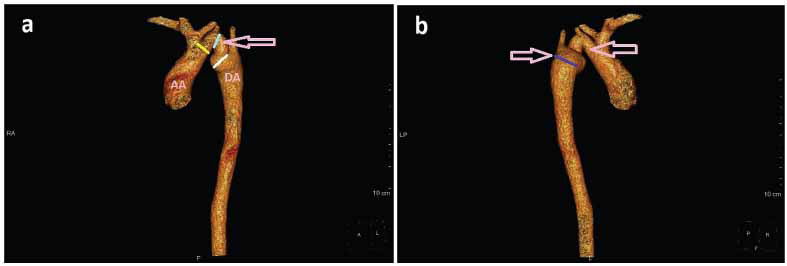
CT-image: 3-dimensional reconstruction of the ascending, arch, and descending aorta. The distal portion of the arch shows slightly reduced calibre, with redundancy and buckling. The descending aorta immediately distal to the buckled segment shows mild prominence in calibre, (a) Yellow line-Proximal arch measuring 2.6 cms, Light blue line-calibre of the kinked segment 2.4cms, and white line- the calibre of the distal portion of the aortic arch, 2.7cms. The pink arrow shows the kinked segment of the aortic arch, and (b) the deep blue line indicates the mildly prominent proximal portion of the descending aorta, 2.8cms, just distal to the distal portion of the kinked aortic arch. The pink arrows show the kinked, buckled aortic arch.
